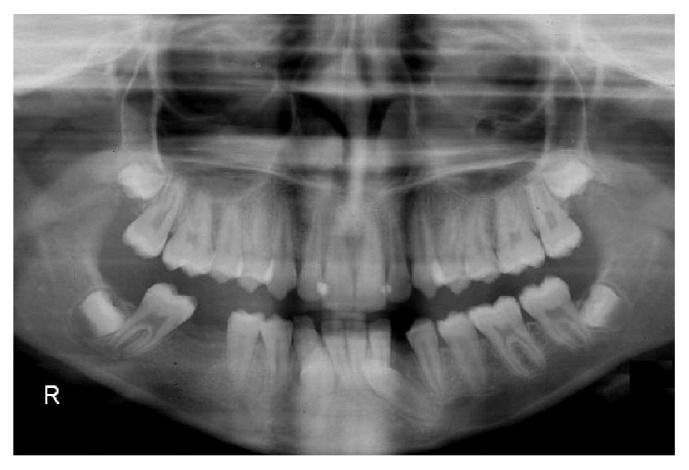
Pretreatment orthopantomograph.
